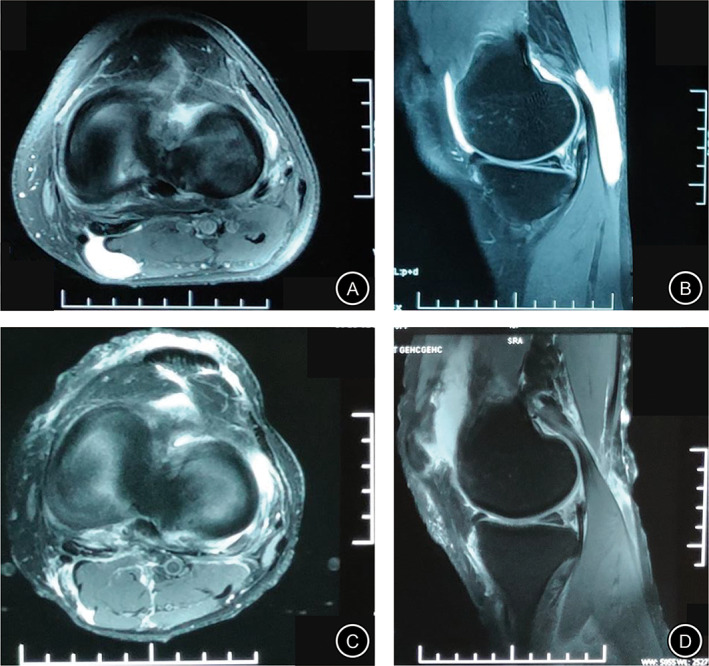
(A & B) Preoperative MRI scan of the axial and sagittal T2 views identifying a popliteal cyst on the left knee of a 55‐year‐old male patient hospitalized for swelling and pain of the left knee for 12.5 months. (C & D) MRI scan of the axial and sagittal T2 views at the last follow‐up examination 22 months after surgery demonstrate disappearance of the popliteal cyst on the left knee.
